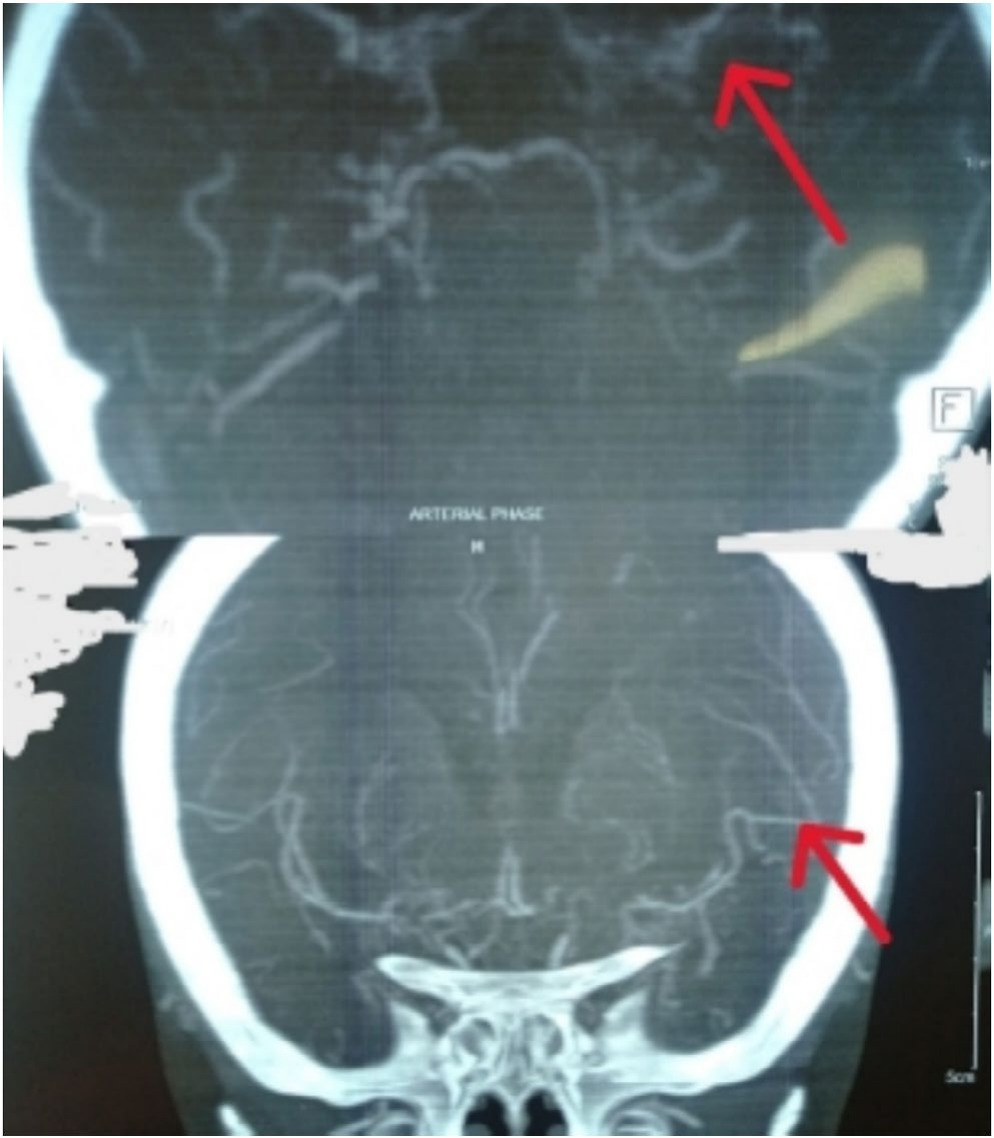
CT scan revealing ill‐defined hypodense area in left fronto‐parietal lobe and right frontal lobe.
